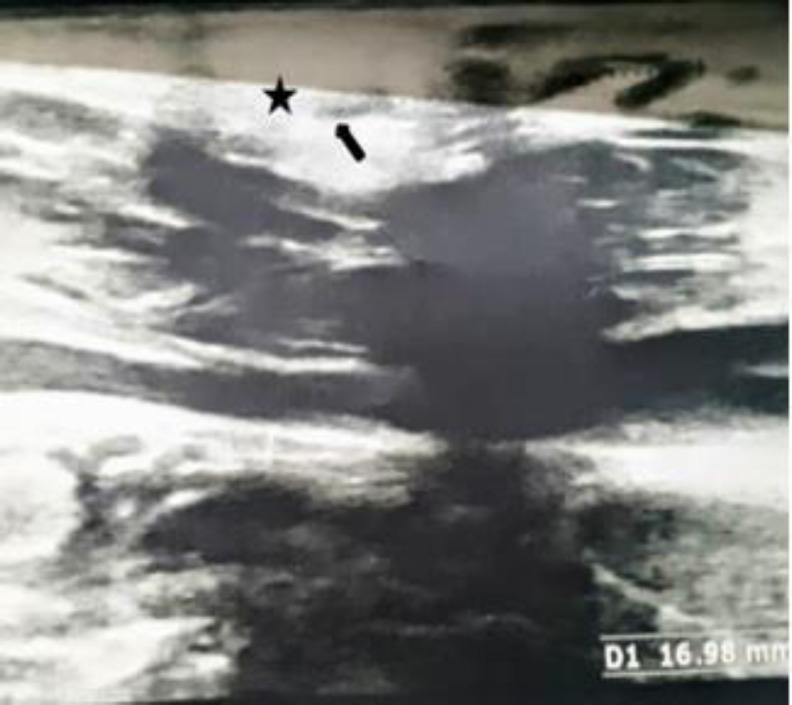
Sonographic findings showing the lesion (the asterisk) and sinus tract (the arrow)
